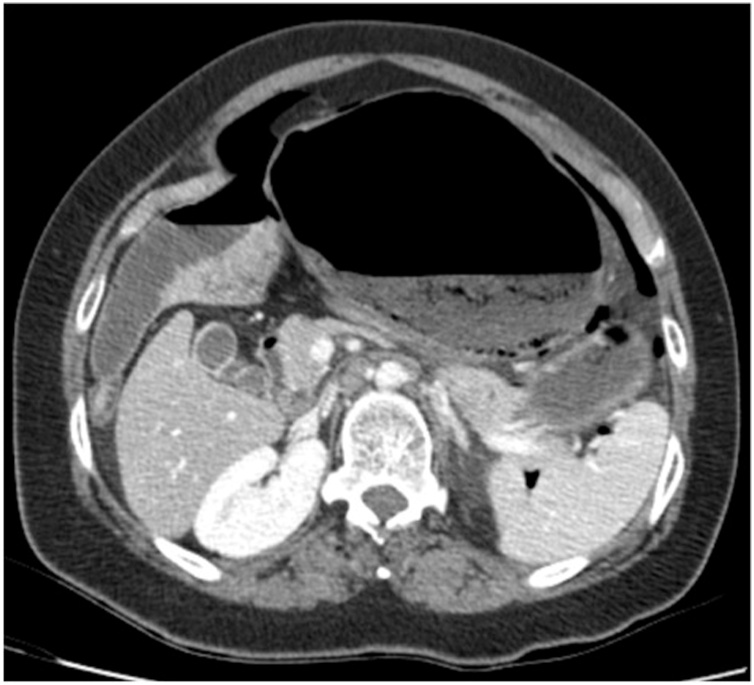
Abdominal CT scan showing a grossly distended cecum in the upper left quadrant.
